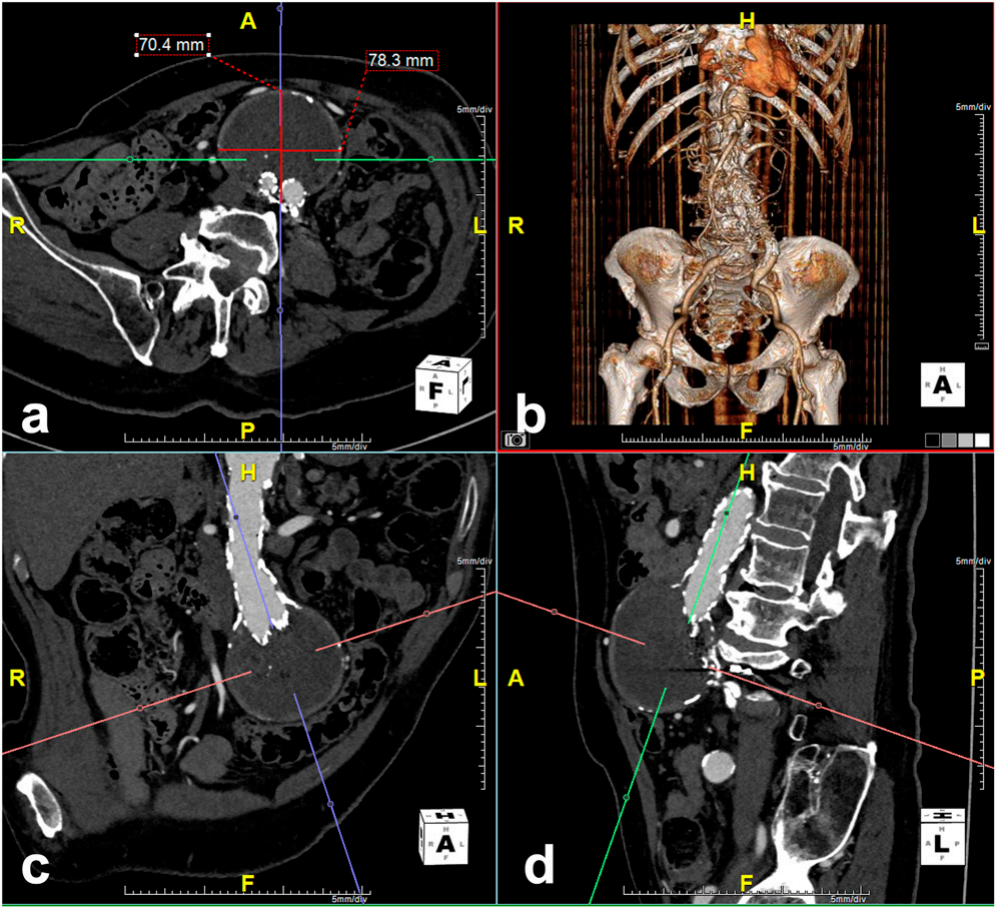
77-year-old man with previous EVAR in 2014. Follow-up CTA in 2016 demonstrated T2EL from a lumbar branch (not shown) and increased aneurysm sac diameter by 11 mm. Aneurysm sac measurements were performed using a three-dimensional post-processing software (TeraRecon, Foster City, California). Anteroposterior (AP) and transverse (TR) measurements of the aneurysm sac (measurements in a) were obtained in the true axial plane (red line in c and d) after adjustments made in the sagittal (blue line in a and b) and coronal (green line in a and c) planes to correct for the tortuosity and angulation of the aorta.
